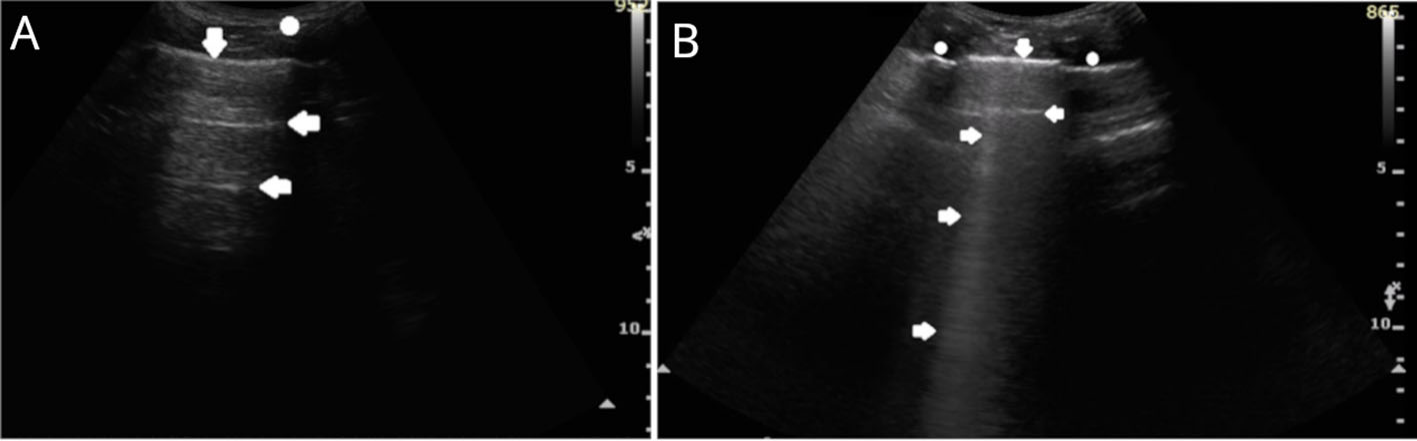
Image of normal lung on ultrasonography. (A) structures of chest wall (o), smooth and regular pleural line (↓), A lines, horizontal artifacts observed in properly aerated lung (←). Convex probe (1-6MHz). (B) ribs and anechoic shadow behind them (o), smooth and regular pleural line (↓), A line artifact (←), B line, vertical artifact of comet tail, in some objects visible in the last intercostal space as a normal variant (→). Convex probe (1-6MHz).
